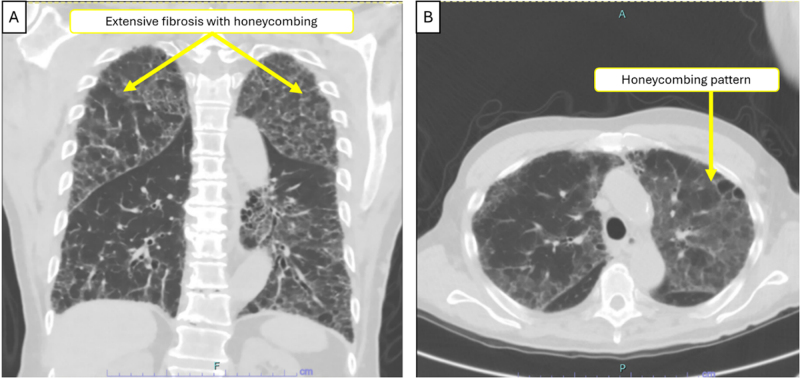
CT chest [frontal (A) and axial sections (B)] showing pre-existing extensive pulmonary fibrosis with honeycombing (arrows).
